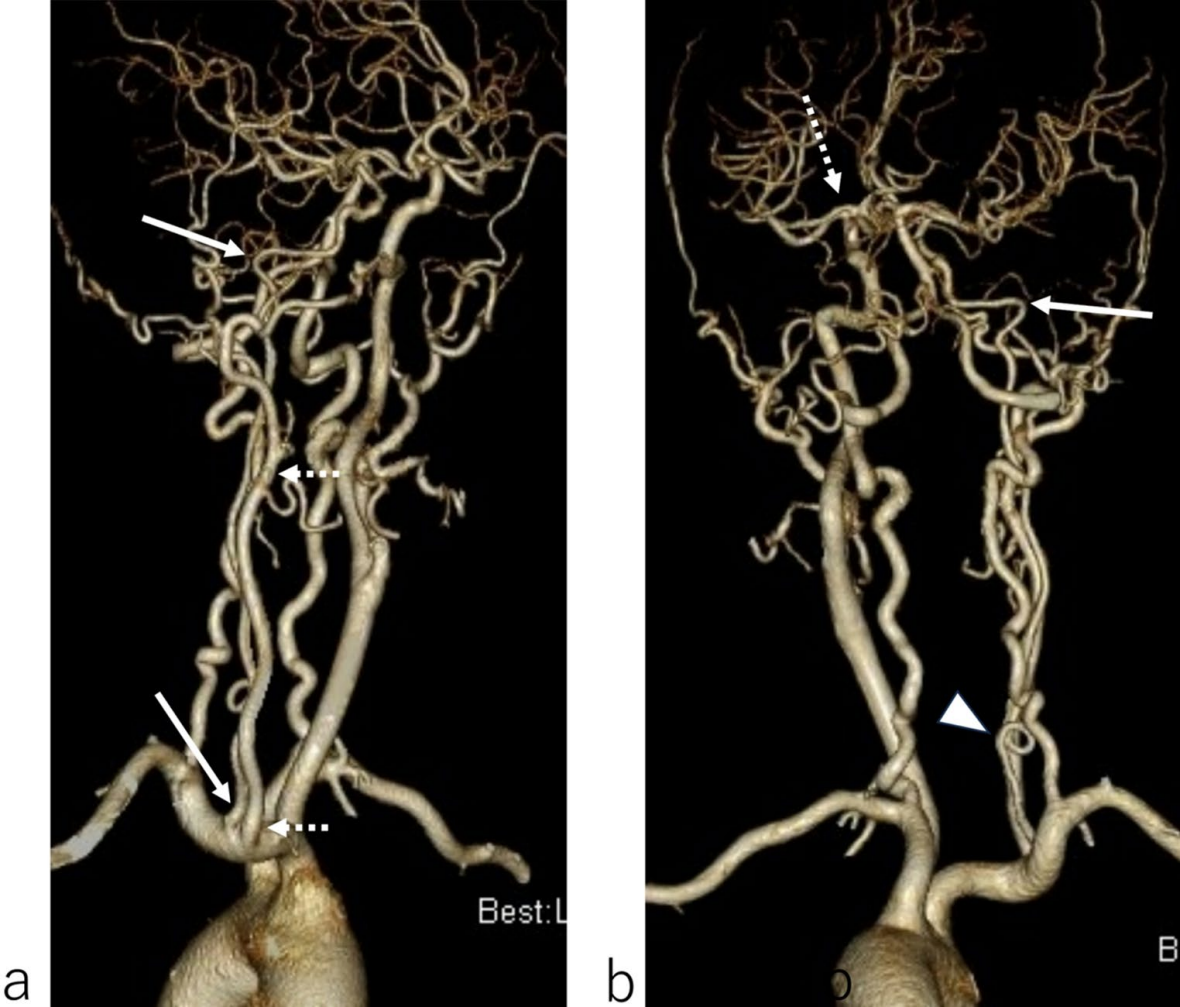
a: Right anterior oblique projection of computed tomography angiography (CTA) shows an absent right common carotid artery (CCA). The right external carotid artery (ECA) branching from the brachiocephalic trunk (BCT) (*dotted arrows*), and the right internal carotid artery (ICA) branching from the right subclavian artery (SA) (*arrows*). b: Slightly left posterior oblique projection of CTA shows that the right ICA is hypoplastic (*arrow*) and forms a coil (*arrowhead*). A left accessory middle cerebral artery (MCA) (*dotted arrow*) is also seen
